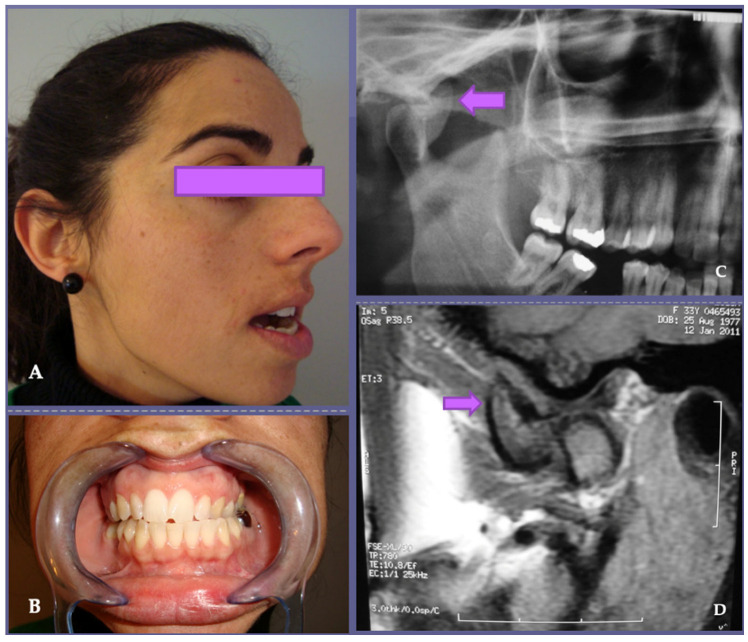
Osteochondroma is one of the most common benign tumors of the axial skeleton but is rarely found in the TMJ. (A) Its clinical presentation in the mandibular condyle, as in our 5th case, usually occurs with a combination of preauricular pain, mandibular dysfunction and facial asymmetry. When considering surgical risks involved in tumoral exeresis and temporomandibular reconstruction, the differential diagnosis is of great importance. (B) In this patient, an osteochondroma was suspected given the clinical characteristics of the lesion; restricted mouth opening and changes in occlusion with unilateral posterior open bite and contralateral crossbite were related to the anatomical location of the tumor and were due to alterations in the vertical dimension. Orthopantomography (C) and magnetic resonance imaging (D) showed an osteochondroma with growth arising from the anterolateral aspect of the right mandibular condyle (see arrows), distinguishing it from condylar hyperplasia, seen as an enlargement of the condylar process.
